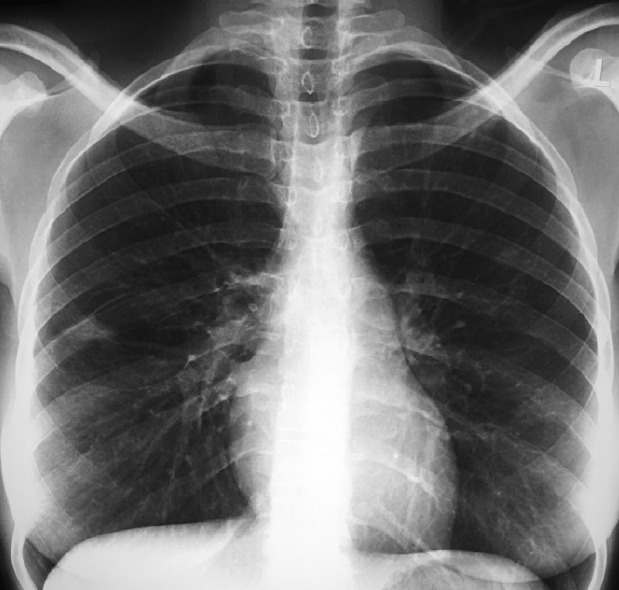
Chest X-ray at completion of treatment.
